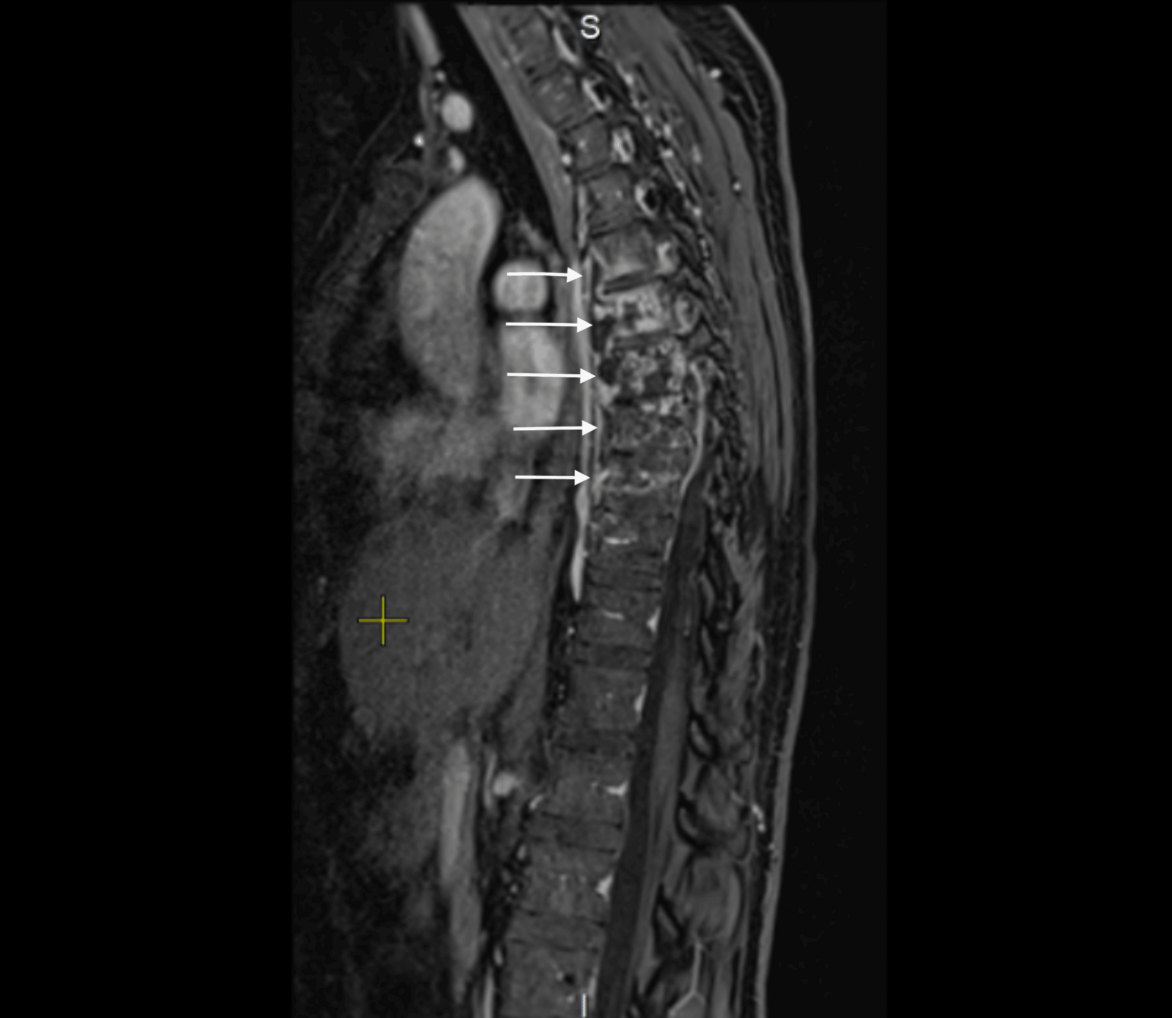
Sagittal MRI of the thoracic spine showing destructive vertebral lesions. White arrows indicate the affected vertebral bodies. MRI: magnetic resonance imaging
